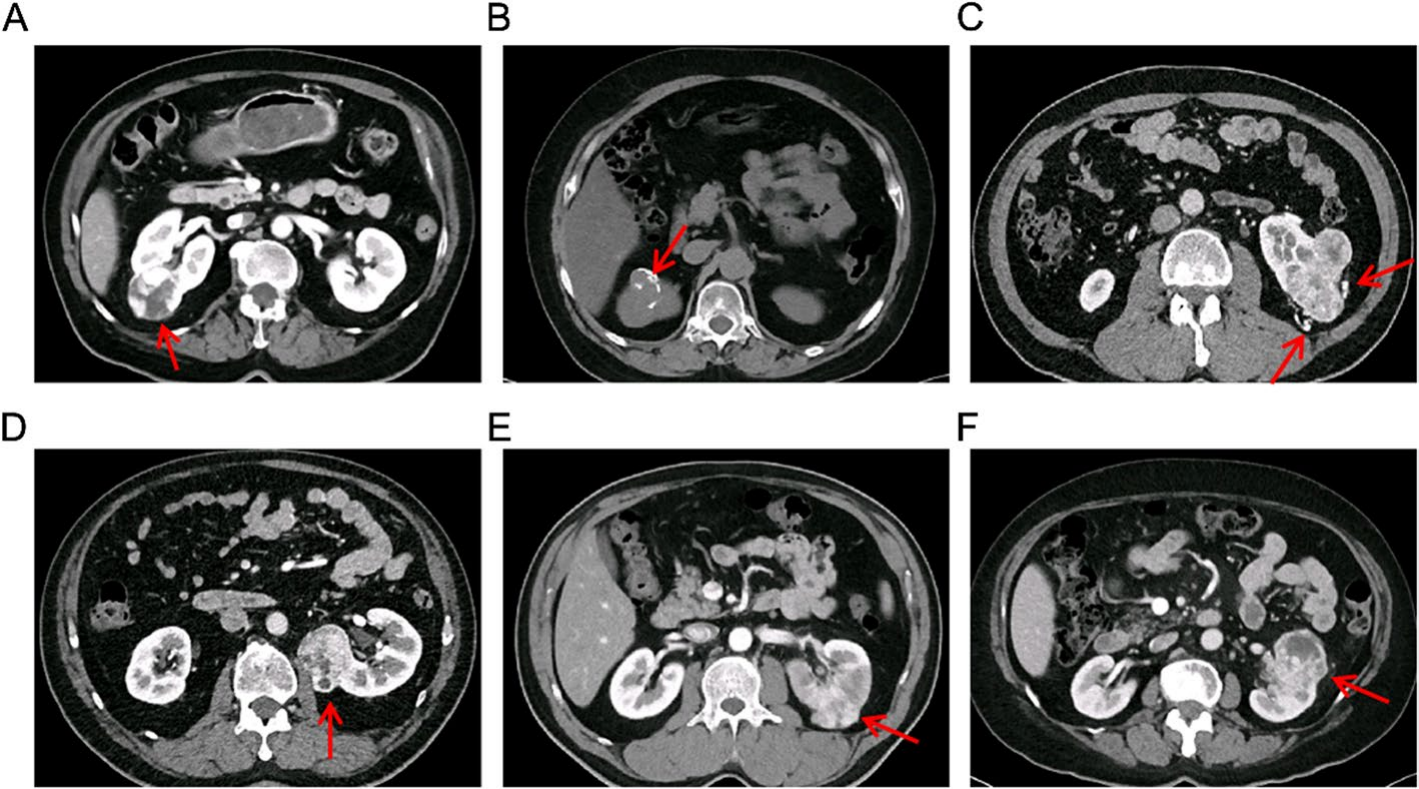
Representative images on CT for cT1 solid RCC: (A) necrosis, (B) calcification, (C) peritumoral neovascularization, (D) tumor margin irregularity: nodular growth pattern, (E) tumor margin irregularity: blurred boundary between renal tumor and parenchyma, (F)tumor margin irregularity: completely non-elliptical shape.  RCC, renal cell carcinoma
